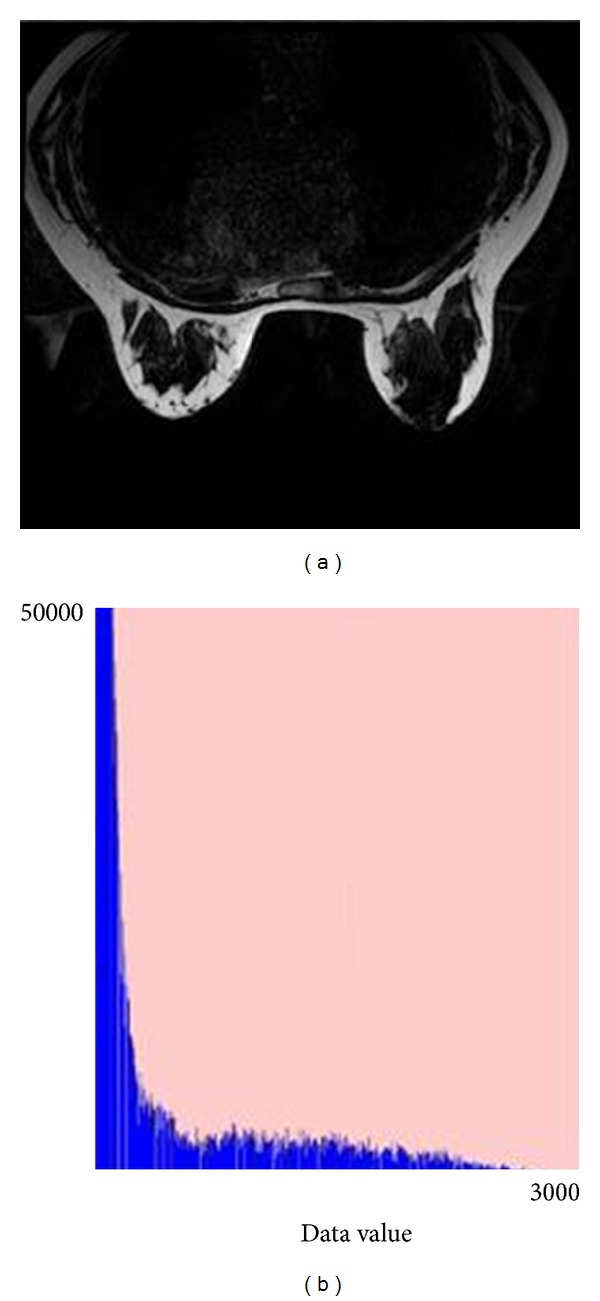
Breast MR image and its histogram. ((a) original MRI; (b) histogram).
